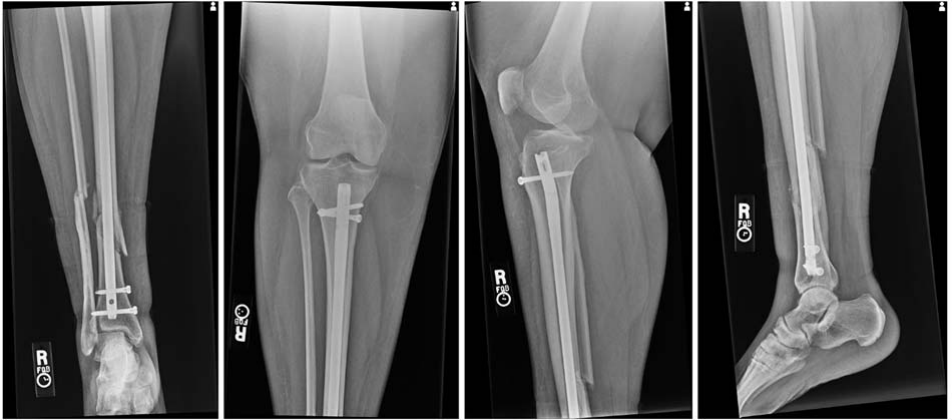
Radiographs at 6 weeks post-op demonstrating unchanged alignment of the distal tibia/fibula fracture.
